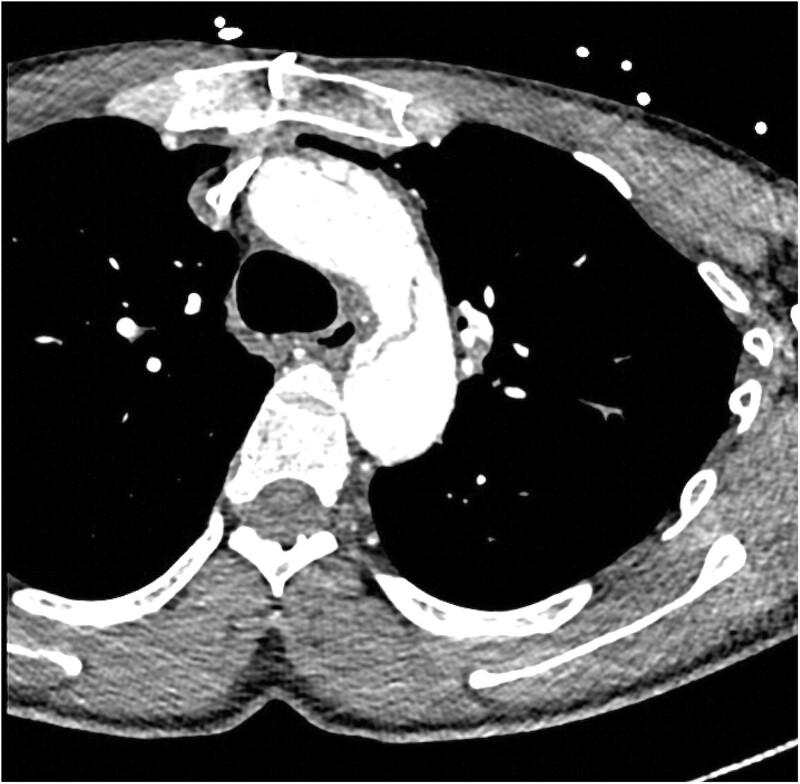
Axial view of a contrast-enhanced CT in arterial phase demonstrating a retrograde type A aortic dissection with patent proximal false lumen in the ascending aorta.
